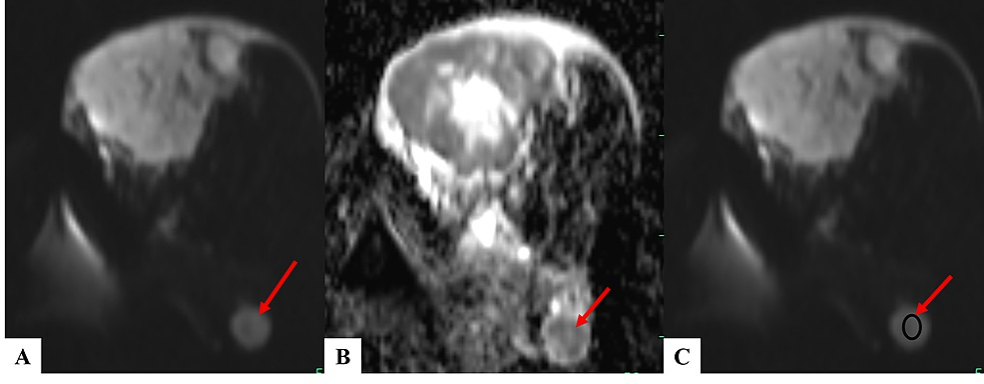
DWI and ADC sequences of the axillary lymph node in a patient with infiltrative ductal carcinoma in the left breast (A) DWI and (B) corresponding ADC images showing a lymph node in the left axilla with restriction of diffusion. (C) DWI image demonstrating method placing a single ovoid ROI on the lymph node for calculation of ADC value. DWI - diffusion-weighted imaging; ADC - apparent diffusion coefficient
